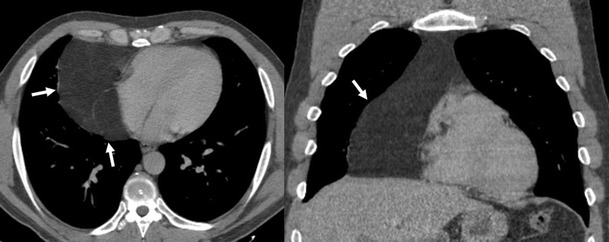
Thymolipoma in a 47-year-old asymptomatic man. Axial and coronal multiplanar reconstruction of non-contrast-enhanced CT scan show a large and well-defined mass (*arrows*) that has extensive fat content and contains small amounts of thin fibrous septa
